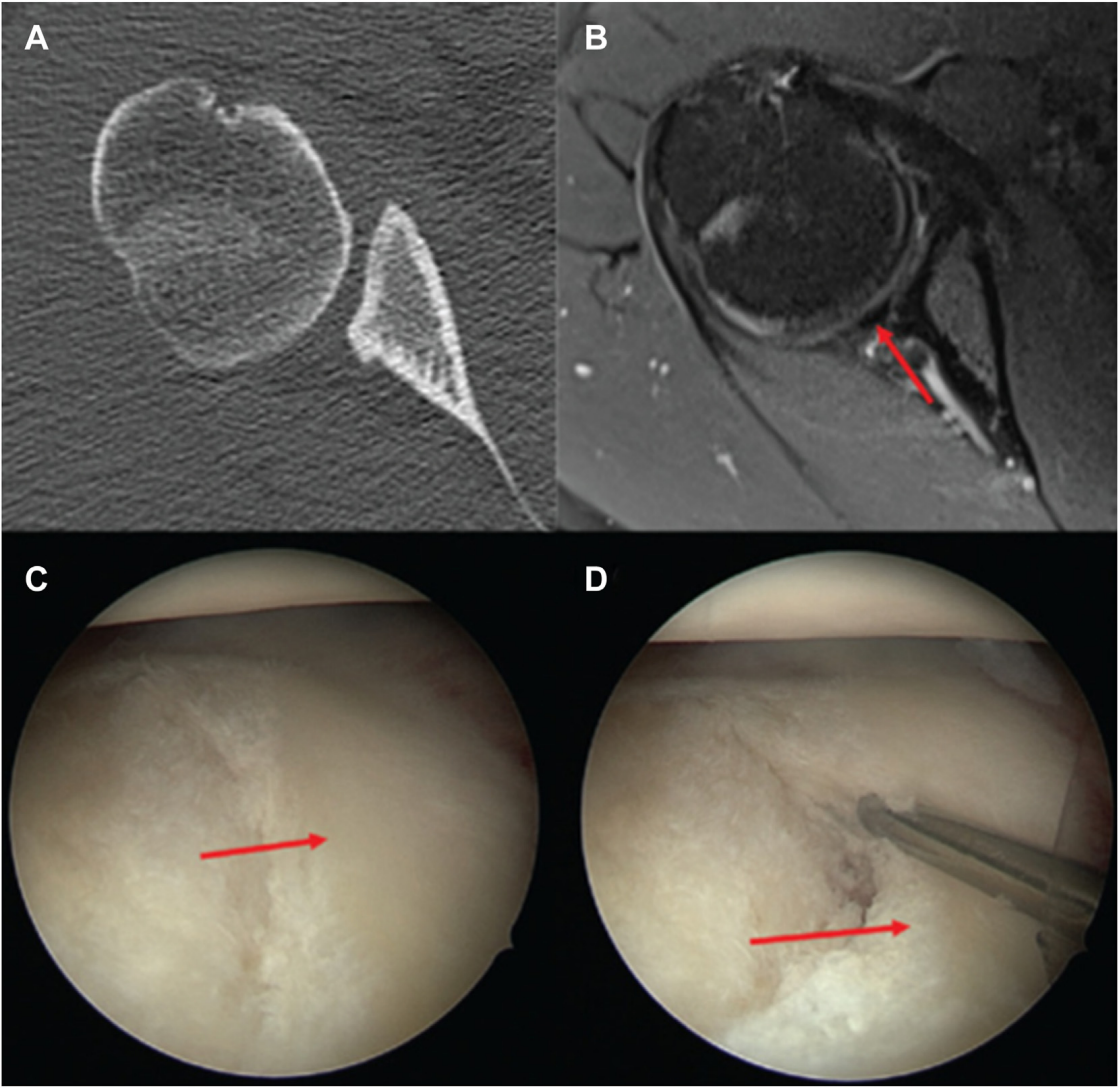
(A) Axial CT demonstrating marked glenoid retroversion and a deficiency of the posterior glenoid rim. (B) MRI scan revealing a compensatory hypertrophy of the posterior labrum (*red arrow*). (C and D) Arthroscopic views of a right shoulder with C1 PSI showing pronounced glenoid retroversion and loss of posterior bony concavity, which is offset by a hypertrophic posterior labrum (*red arrows*). Figure reused with permission from Moroder et al^29^. *CT*, computed tomography; *MRI*, magnetic resonance imaging; *PSI*, posterior shoulder instability.
